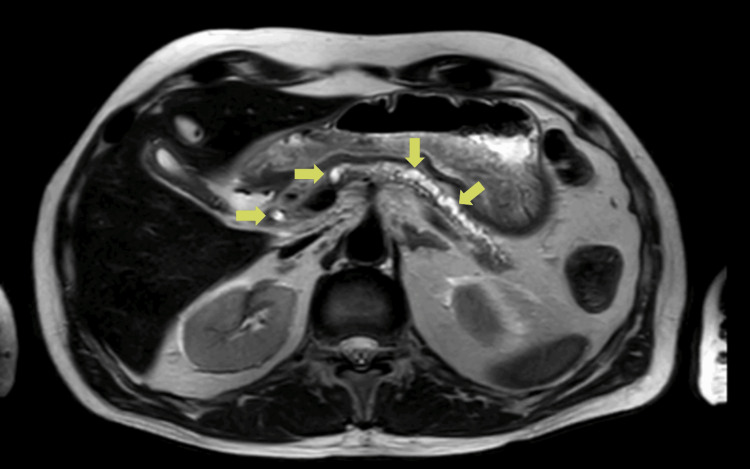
Preoperative MRI Multiple liths are observed along the Wirsung duct (marked with green arrows).
